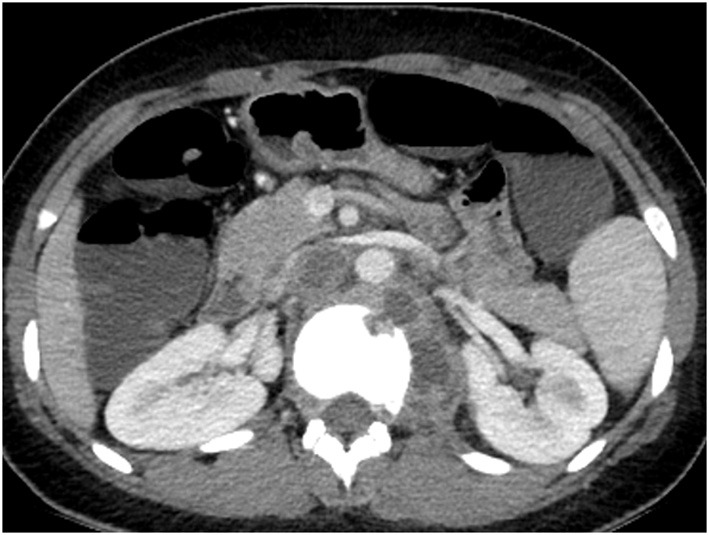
Abdomen CT conducted at 3 months after cessation of miliary TB treatment. A newly defined presumed cold abscess within the left psoas muscle at the level of T10 to L2 and TB spondylitis at the T12 and L1 are observed. CT, computed tomography; TB, tuberculosis.
